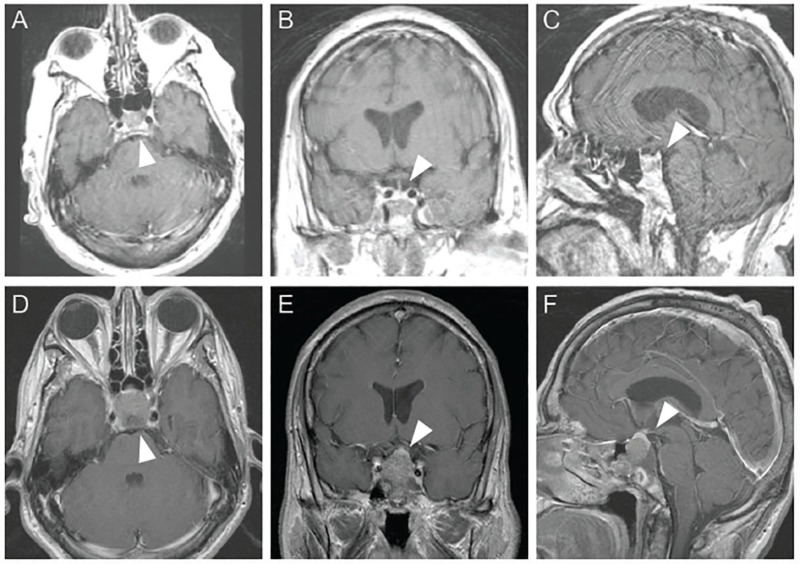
The patient presenting with Cushing disease Gadolinium-contrasted T1 brain MR images in axial (A), coronal (B) and sagittal (C) planes demonstrating no clear evidence of a microadenoma and showing possible fibrous dysplasia or other benign fibro-osseous lesion. Due to clinical deterioration, the patient underwent repeat MRI one year later revealing a new sellar lesion - axial (D), coronal (E), and sagittal (F). White arrows designate sellar and parasellar region of interest.
